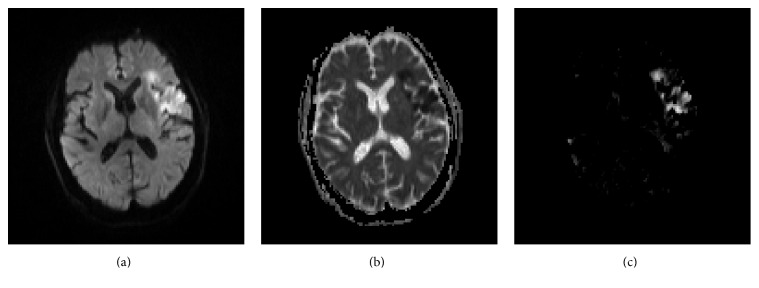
From (a) to (c), an axial slice of DWI and ADC and corresponding asymmetry map ASYM.
